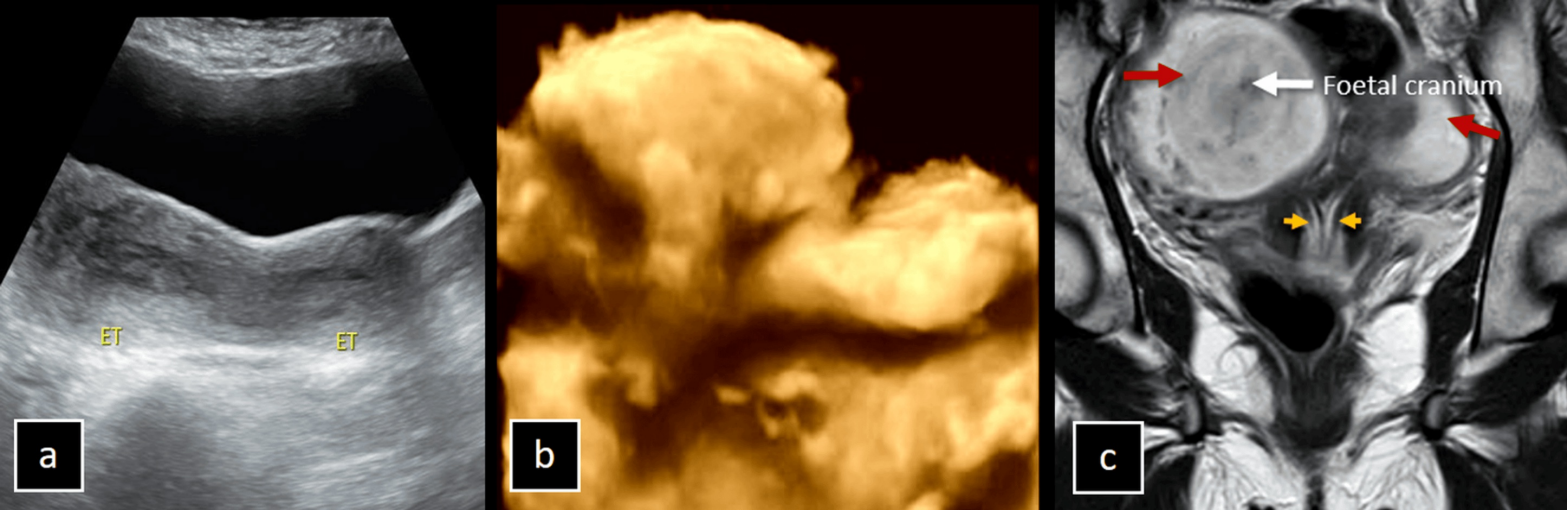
(a-c) Imaging of the Bicornuate Bicollis Uterus in a Patient With Abdominal Pain. Greyscale ultrasound (a) and 3D ultrasound (b) of a 19-year-old female during a routine abdominal ultrasound for evaluation of pain revealed an incidental finding of a bicornuate uterus with two separate cervices. Further MRI, T2 coronal section (c) during the antenatal period, demonstrates two divergent uterine horns (red arrows) with a solitary vagina but separate cervices (yellow arrowheads), consistent with a bicornuate bicollis uterus. A single intrauterine gestation is present in the right horn.
